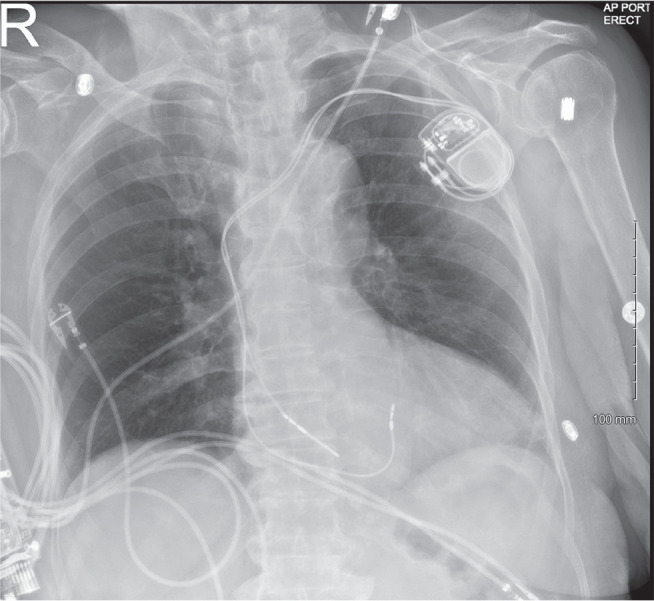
Chest X-ray showing pacemaker with leads implanted in the right atrial appendage and deep into the interventricular septum.
